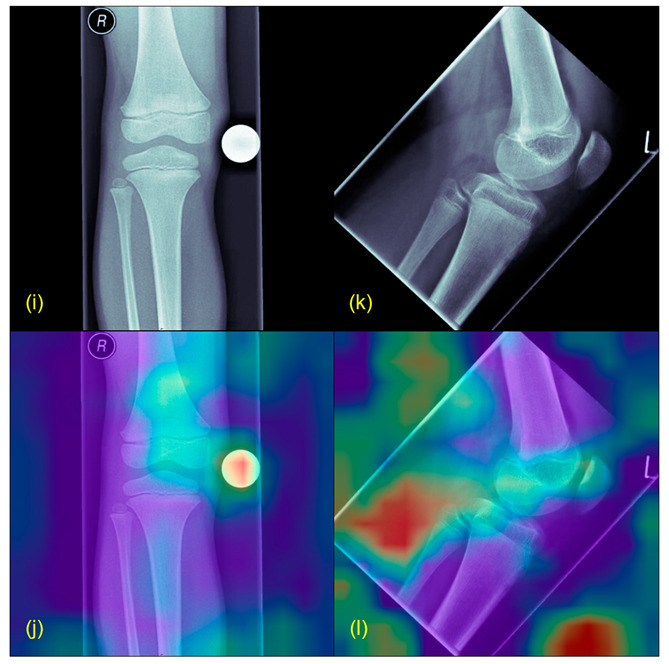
Grad-CAM example from misleading results. (i,k) show the original X-rays, (j,l) the Grad-CAMs of the same images. Highlighted in red are the parts wrongly marked as suspicious (for example the scaling ball in (i)).
